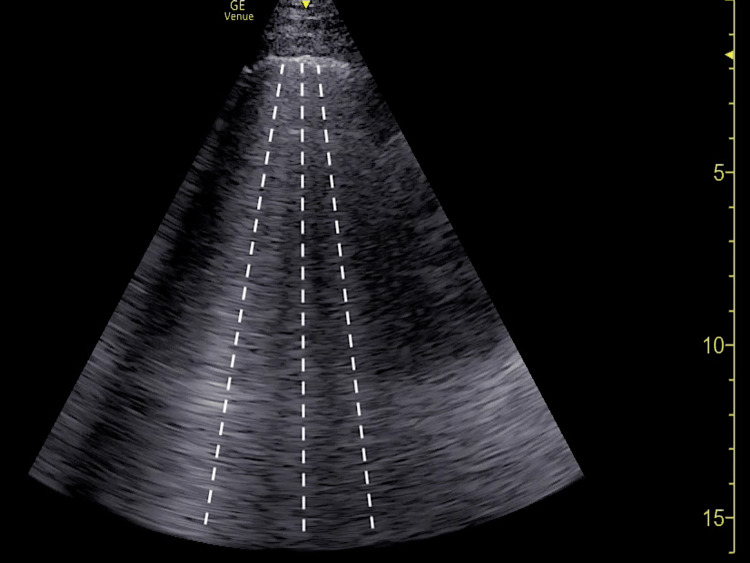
Representative point-of-care lung ultrasound demonstrating diffuse B-line profile (dotted lines) suggestive of multifocal pneumonia versus pulmonary edema.
